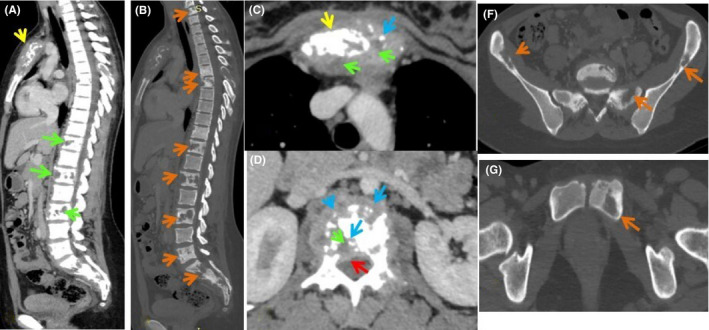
Thoraco‐abdominopelvic CT after contrast injection: Sagittal (A, B), axial (C, D, F, G), parenchymal (A, C, D) and bone (B) windows (F, G): Multiple osteolytic lesions surrounded by peri‐lesional osteo‐condensation of the cervico‐dorso‐lumbo‐sacral spine, iliac wings and left pubis (orange arrows) with rupture of the cortical bone and bone sequestration by location (light blue arrows) real masses in the sternum (yellow arrows) and sacrum (dark blue arrow). Evidence of multiple abscesses intra‐somatic and sternal (green arrows). It associates an epiduritis at L1 level (red arrow)
